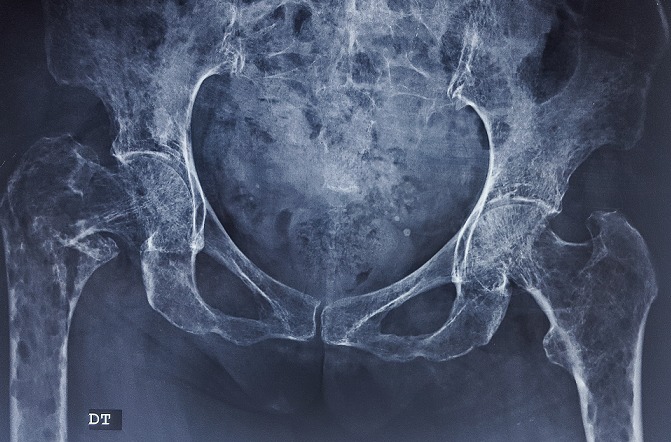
Radiographie de la hanche de face: fracture pertrochantérienne du fémur droit avec un large fragment du petit trochanter; multiples images lacunaires disséminées dans les deux fémurs et les os du bassin
